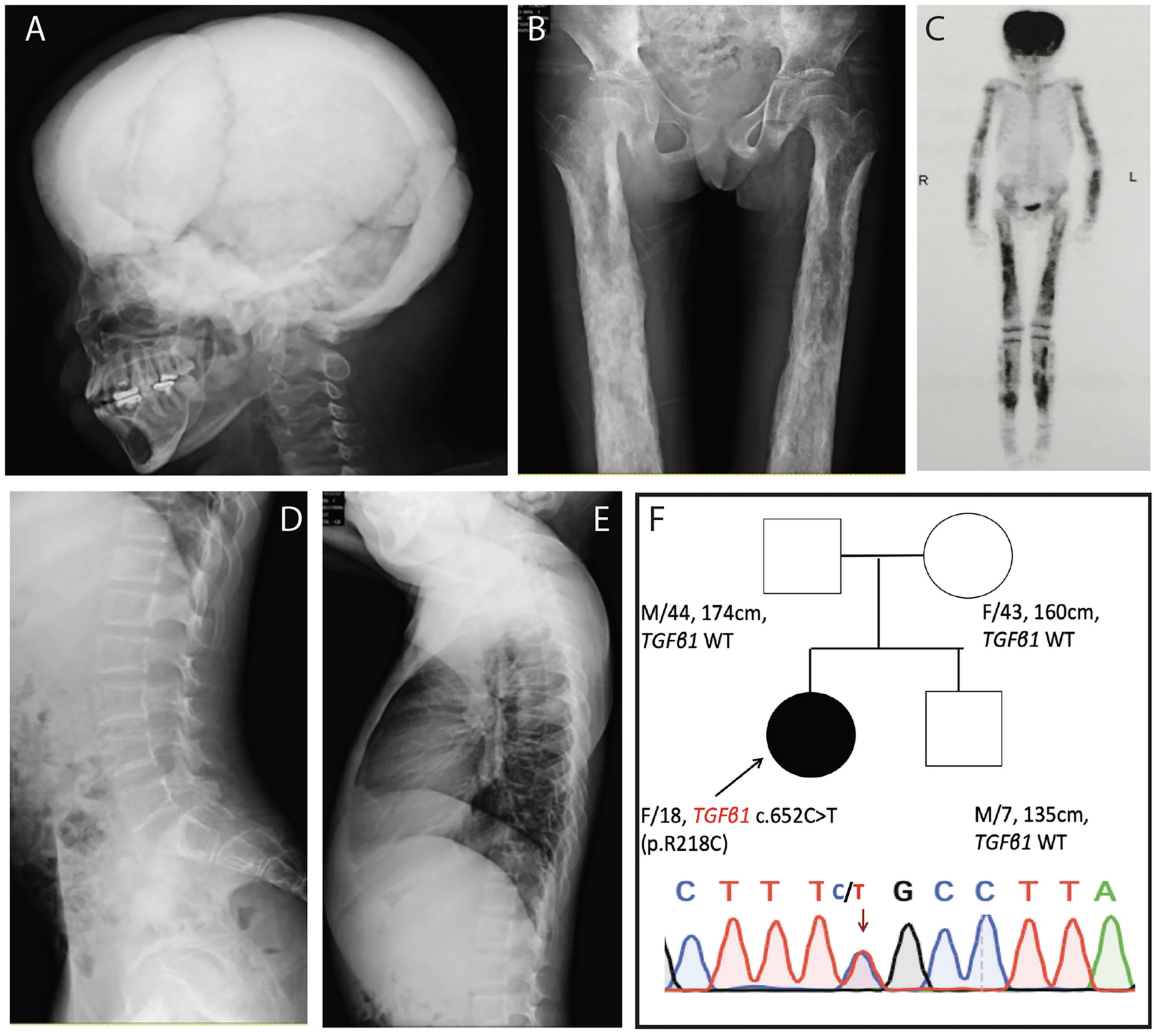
Clinical manifestation of the patient with CED. (A, B) X-ray revealed thickening of skull and thickening of cortices in the diaphysis of both femur. (C) Bone scintigraphy revealed high uptake in skull and the diaphysis of upper and lower limbs. (D, E) X-ray revealed sparse bone trabeculae in thoracic and lumbar spine. (F) Sanger sequencing detected a mutation of c.652C>T (p. R218C) in the gene *TGFB1* (NM_000660) in the patient, but neither in her parents nor her brother.
